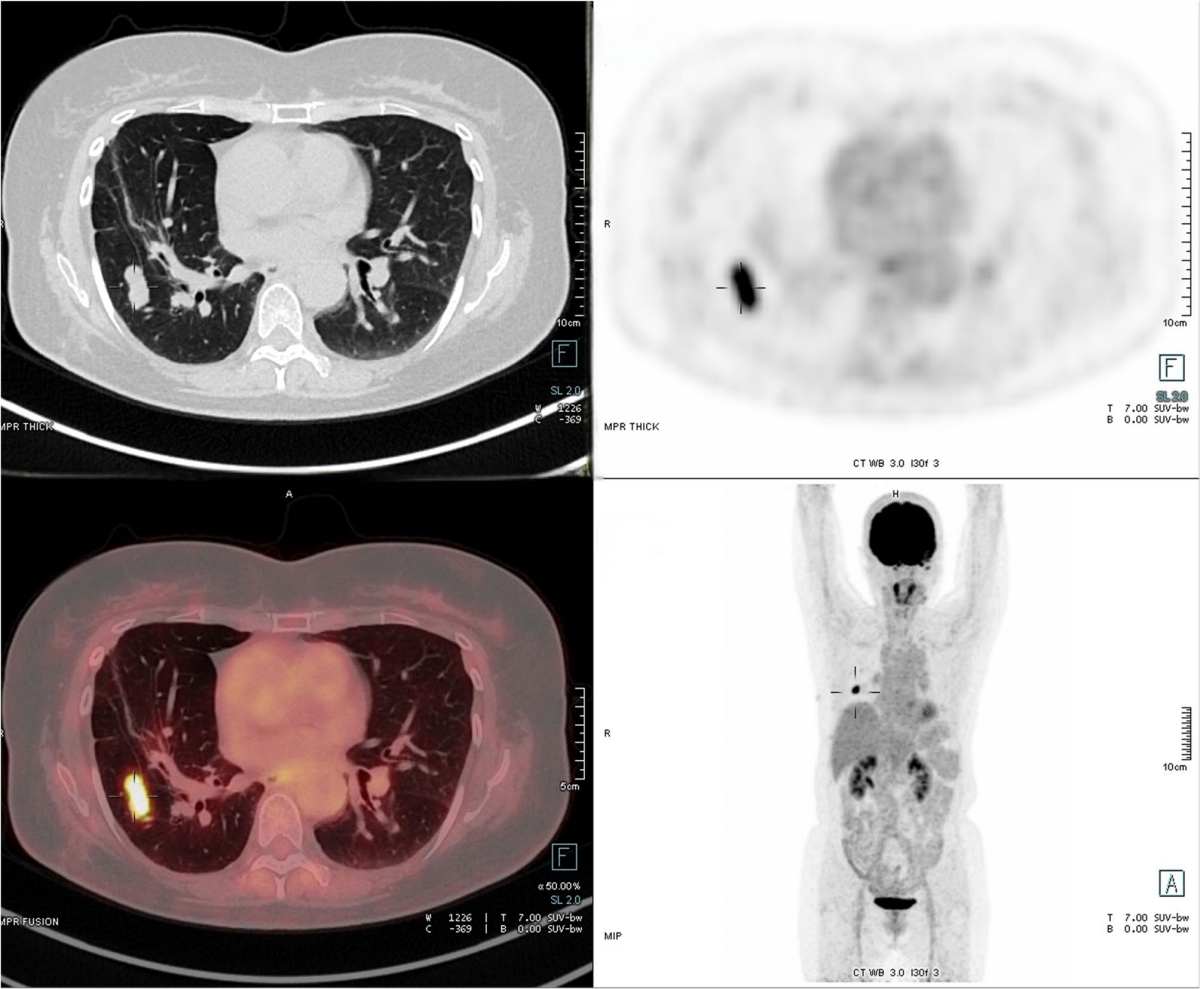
18F-FDG PET/CT revealed an irregular nodular opacity in the right lower lobe with relatively well-defined and smooth margins. The maximum axial dimension measured 2.8 × 1.2 cm, demonstrating intense FDG uptake (SUVmax = 11.4)
